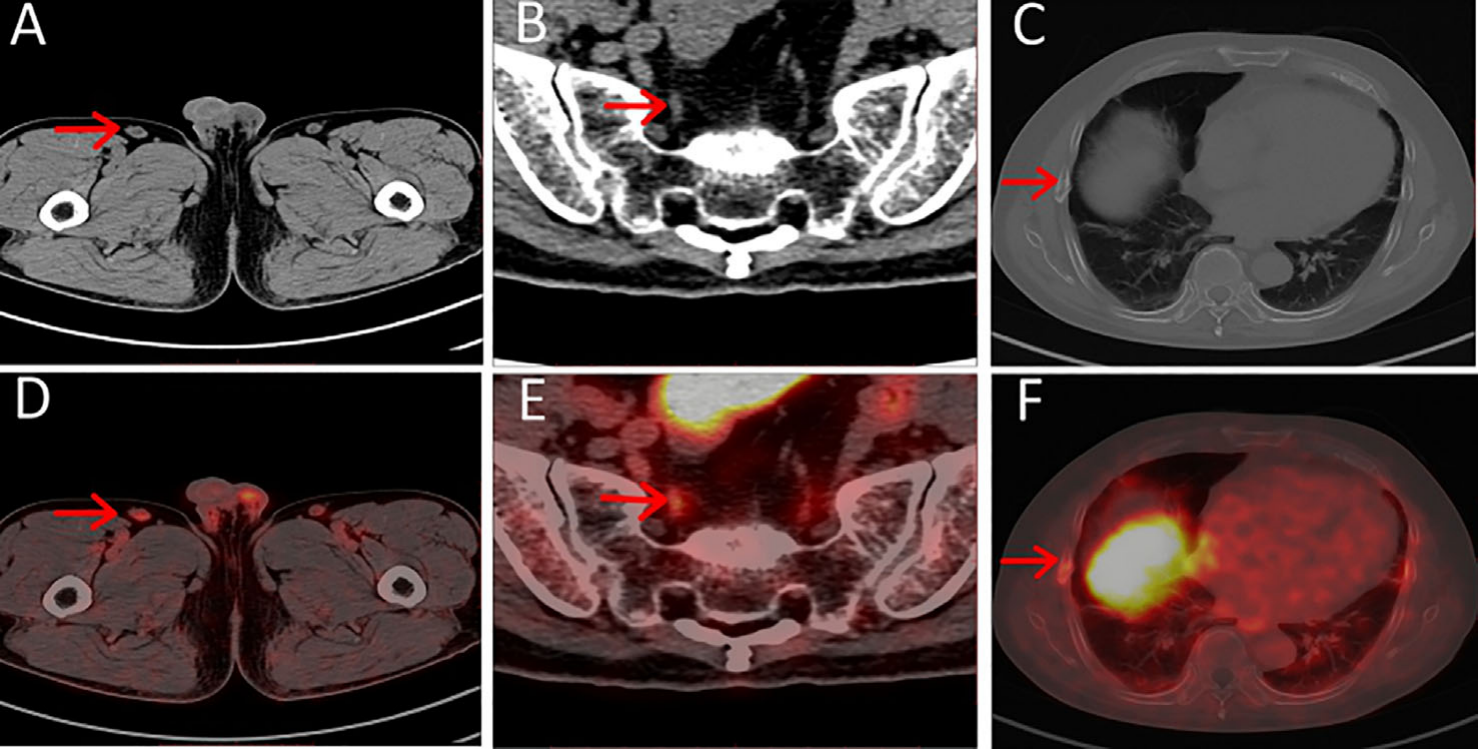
(A–C) Axial CT, (D–F) axial PET/CT of pitfalls in ^18^F-PSMA-1007. Unspecific PSMA-ligand uptake in right inguinal lymph node (A, D), PSMA-ligand uptake in pelvic ganglia (B, E) and PSMA-ligand uptake in right non-displaced rib fracture (C, F).
